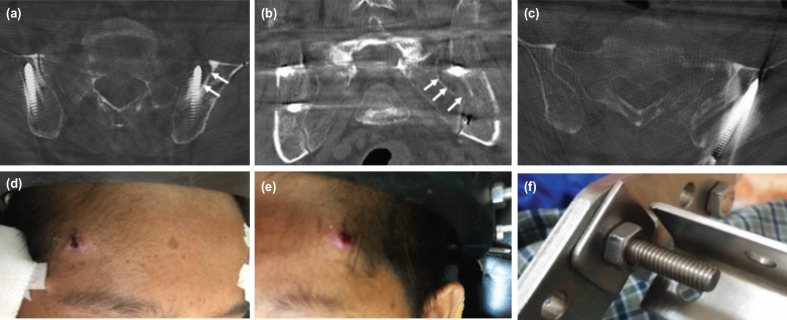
(a, b c) CT pelvis of Case 4 showing septic loosening with osteomyelitis (white arrow). (d, e) Photographs showing loosening and migration of anterior halo pins in Case 2. (f) Showed a broken halo-pelvic strut.
